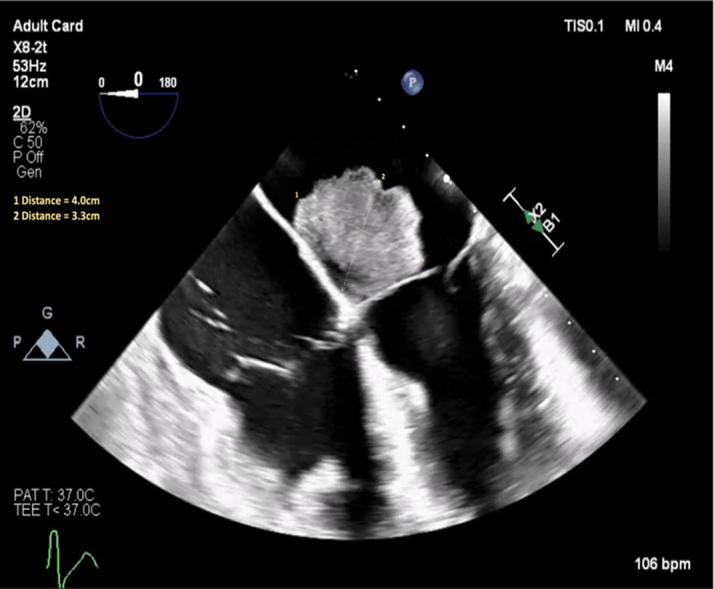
Transesophageal Echocardiography With Multilobulated Atrial Mass
